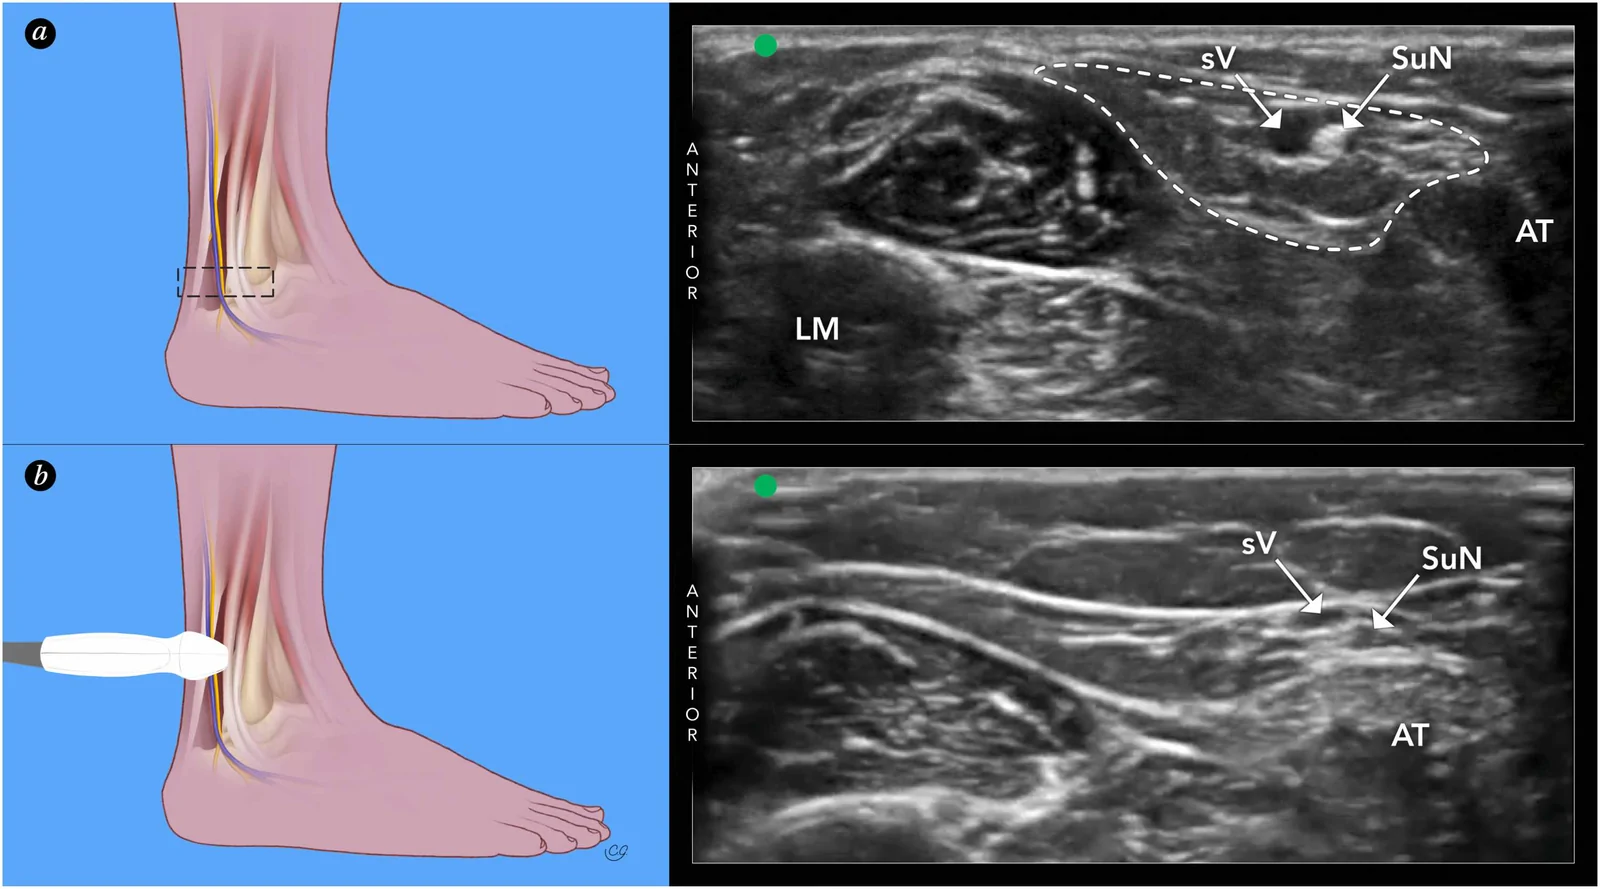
Ultrasound image and probe positions for the sural nerve block. (a) In the distal view, the probe is placed between the lateral malleolus and the Achilles tendon, where the sural nerve is identified within the fascial expansion (arrowheads) adjacent to the lesser saphenous vein. (b) To obtain a clearer view of the sural nerve, the probe is moved proximally and posteriorly. The sural nerve is seen closer and superficial to the Achilles tendon. LM: lateral malleolus, sV: lesser saphenous vein, SuN: sural nerve. Reproduced with permission from Girón Arango Medicine Professional Corporation.
